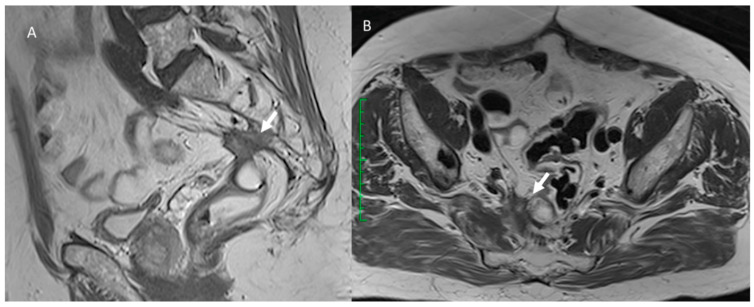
MRI assessment of rectal cancer relapse. In (A) (T2-W in axial plane) and (B) (T2-W in sagittal plane), arrows show relapse that involves rectal wall and external planes.
